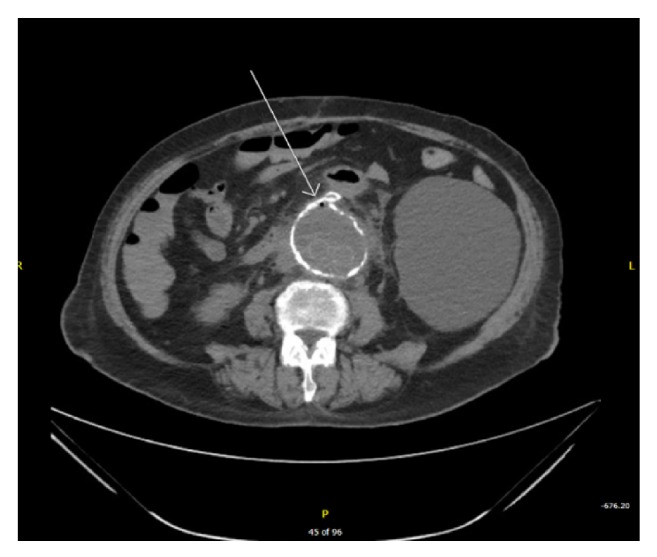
Computed tomography of abdomen showing tiny foci of air at the anterior aspect of the native aneurysm wrap just inferior to the location where the duodenum crosses.
